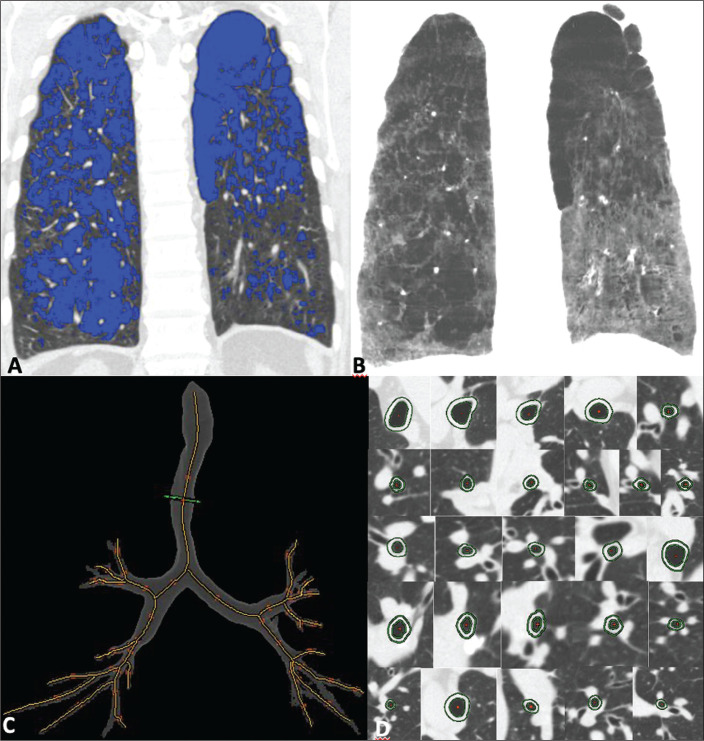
A,B: Quantification of emphysema on chest CT scans. A: Coronal image showing blue areas corresponding to regions with less than -950 HU (emphysema). B: Coronal image with minimum intensity projection reconstruction showing extensive areas of emphysema with low attenuation. C,D: Quantification of tracheal and bronchial wall thickness. C: Three-dimensional reformatting with lines on the central regions of the bronchial segments analyzed. D: Example of transversal plane measurement for each of the 27 segments (trachea, plus first, second, and third generation bronchi).
